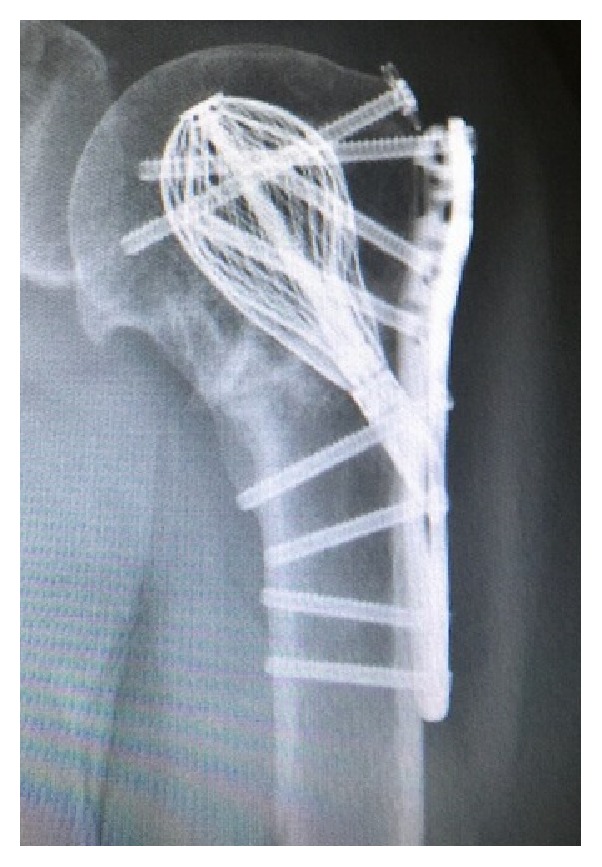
Radiograph at 6-month follow-up after fixation using a PH Cage and side plate construct.
